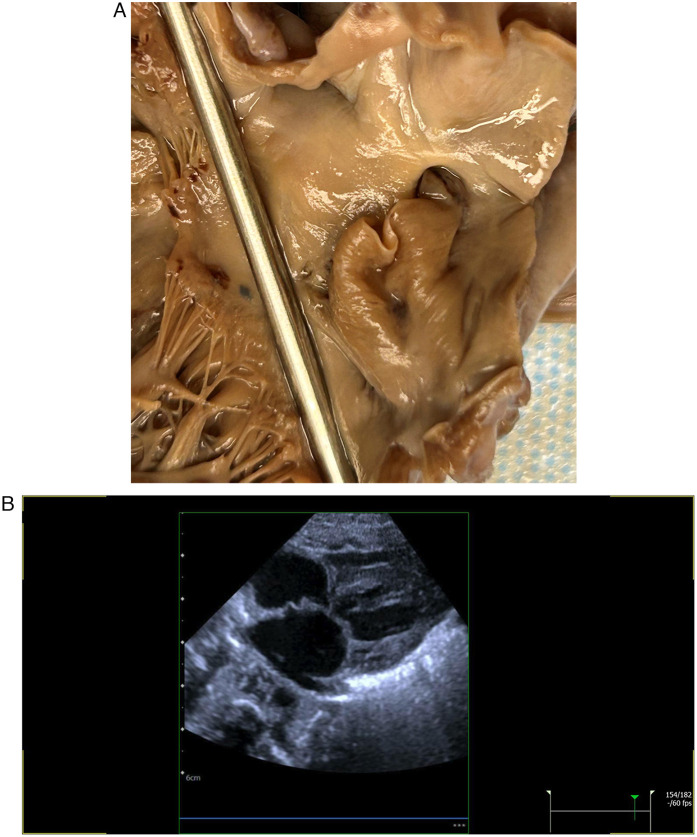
(A) Autopsy photograph, left side of the interatrial septum of a thick, aneurysmal interatrial septum, with a small, ectopic opening. (B) Transthoracic echocardiography, subcostal four-chamber view, of the thick interatrial septum depicted in (A).
